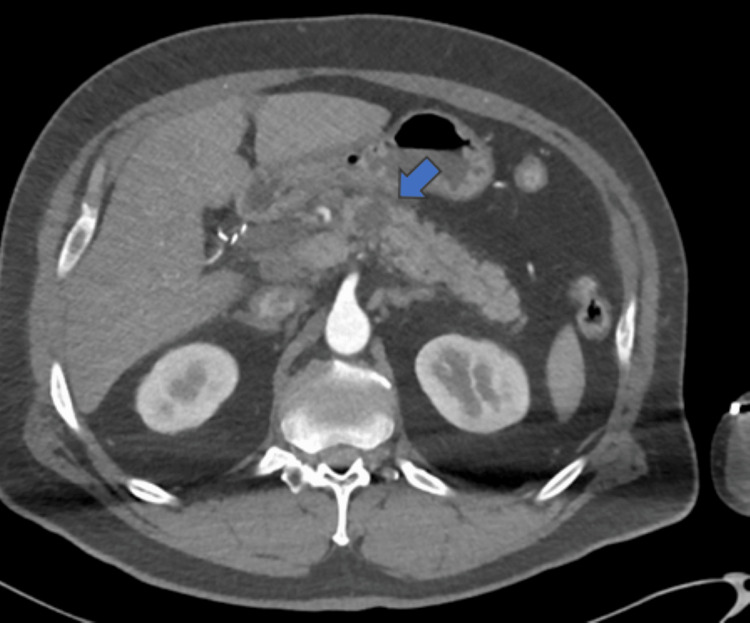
Computed tomography scan of the abdomen on first admission with a 1.8 cm hypoattenuating mass (blue arrow), identifying a possible pseudocyst, in the pancreatic body with related adjacent edema and fat stranding.
